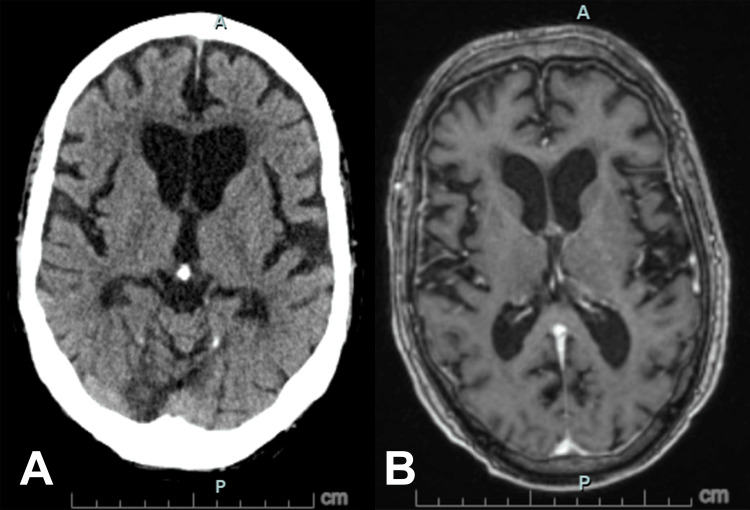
Axial non-contrast CT (A) and post-contrast T1-weighted MRI (B) of the brain showing no acute intracranial abnormality, with age-related changes noted.
